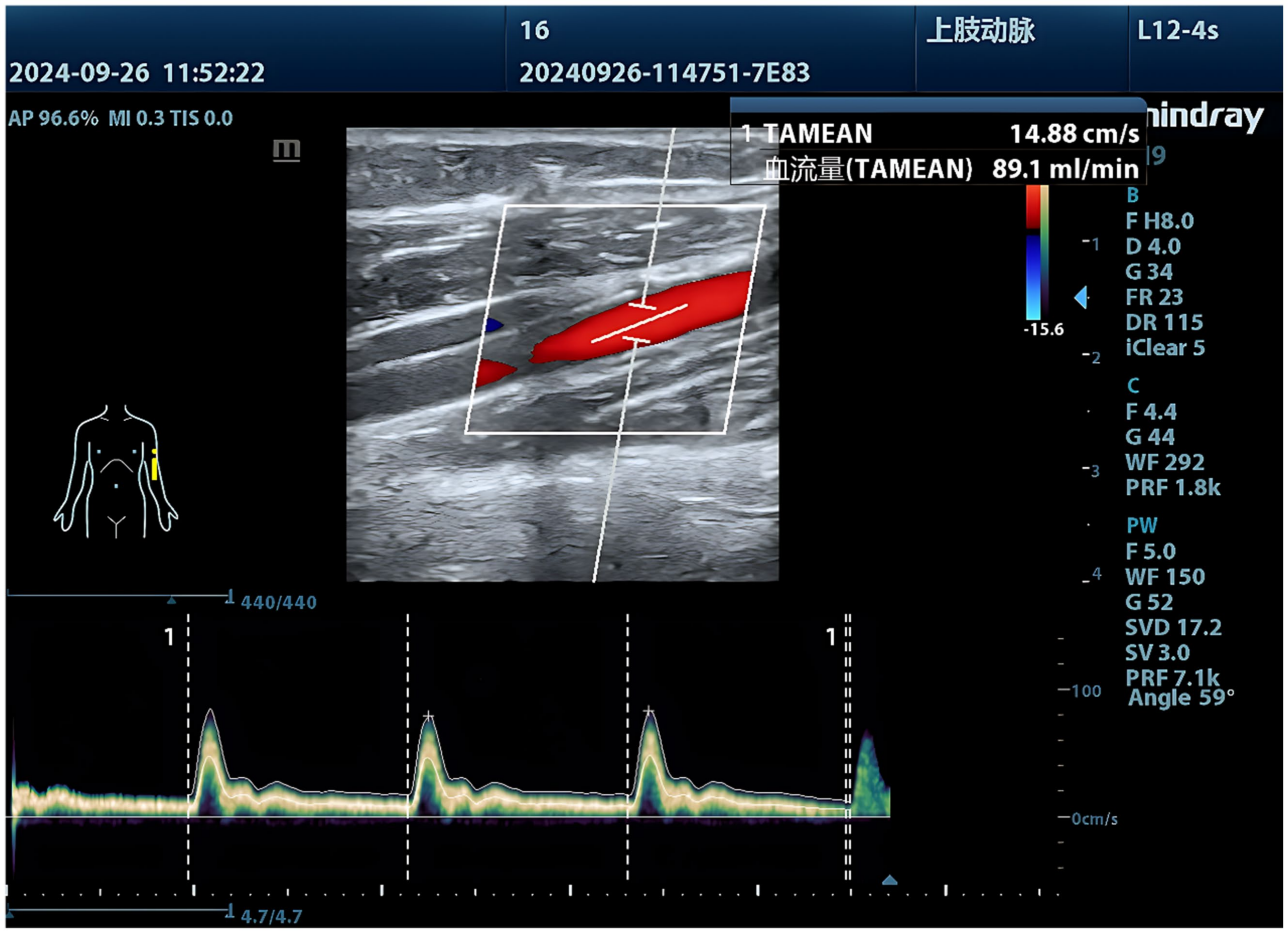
Portable color Doppler ultrasound displays arterial blood flow.
